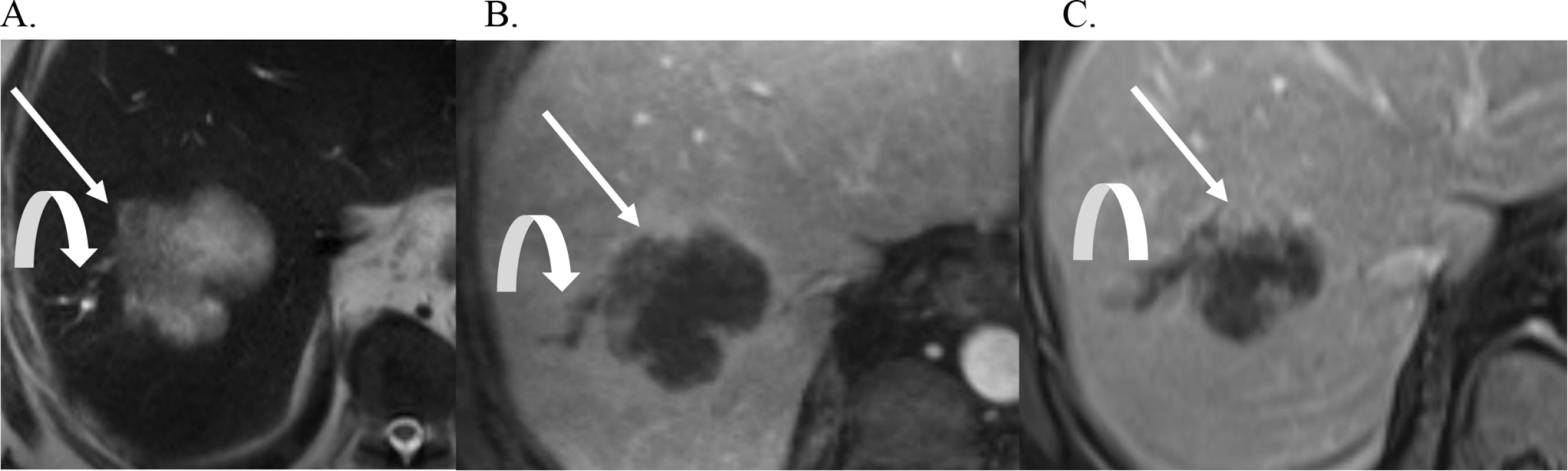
Intrahepatic Cholangiocarcinoma. Axial T2 (A), and post contrast T1-weighted portal venous phase (B) and delayed phase (C) images demonstrate a 6.1 cm mass forming CCA (arrow) in hepatic segment 7. There is rim enhancement on the portal venous phase (B) with centripetal central enhancement on the delayed phase (C). There are dilated upstream bile ducts peripheral to this mass (curved arrows)
